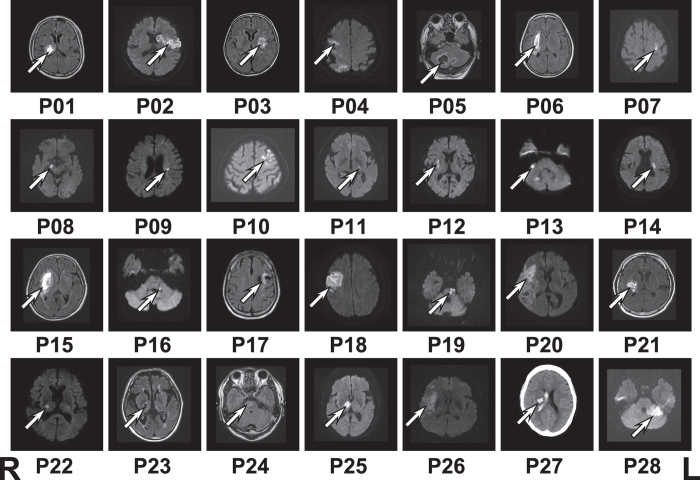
Lesion location of each patient. Patient number is according to modality dominance of motor imagery, P01 to P8 for kinesthetic dominance and P9 to P28 for visual dominance. Lesion locations were shown by fluid attenuated inversion recovery (FLAIR) MRI scans for hemorrhagic stroke (P01, P03, P05, P06, P15, P21, P23, and P24) and by diffusion-weighted image (DWI) MRI scans for ischemic stroke (P02, P04, P07−P14, P16, P18−P20, P22, P25, P26, and P28). Lesion location of P17 with ischemic stroke was shown by FLAIR MRI scan. Lesion location of P27 with hemorrhagic stroke was shown by CT scan. Left side of the figure represents the right side of the brain.
